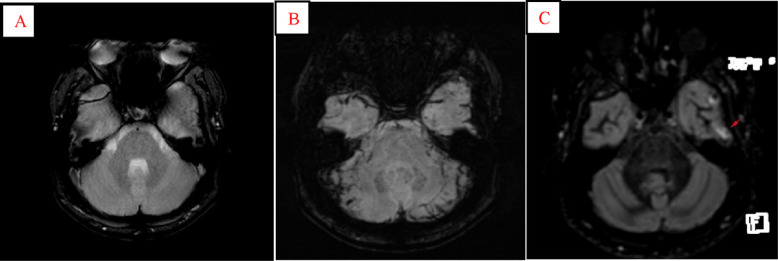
SAH in temporooccipital subarachnoid space in a 32-year-old man who done MRI 17 days after traumatic injury. In axial T2* (A) and SWI (B) images, no signal abnormality is detected because of the destructive susceptibility artifact from air-tissue interfaces in the temporal lobes. Conversely, distinguished signal intensity in DIR (C, arrow) image is observed.
